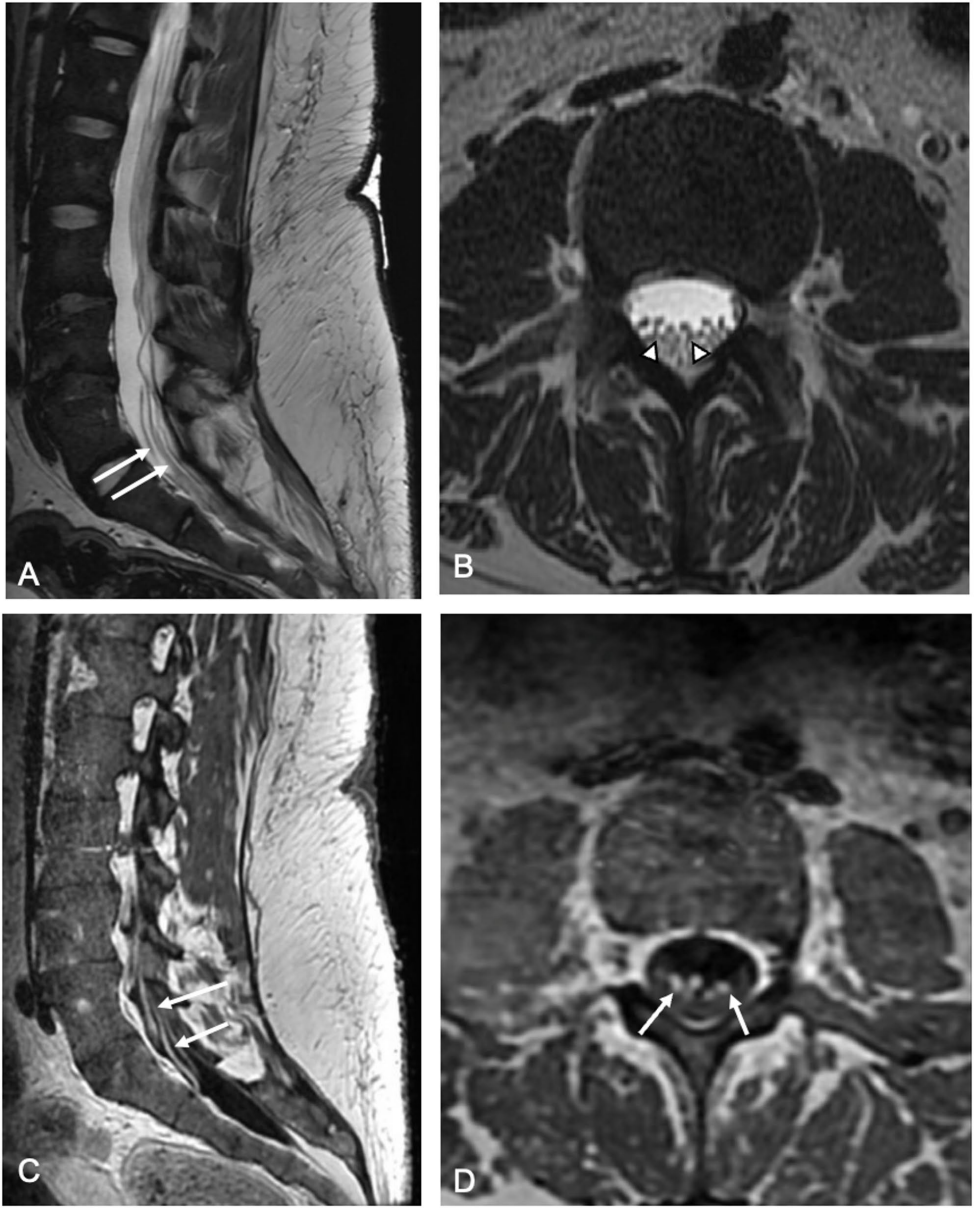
Cauda equina polyneuritis at day 13 after CAR T-cell infusion in a 25-year-old woman with acute lymphoblastic leukemia and subacutely worsening weakness of lower extremity weakness. (A) Sagittal and (B) axial T2-weighted MR images of the lumbar spine demonstrate thickening of multiple cauda equina nerve roots (white arrows and arrowheads). C) Sagittal and D) axial contrast-enhanced T1-weighted MR images demonstrate enhancement of the thickened nerve roots consistent with diffuse polyneuritis (white arrows)
